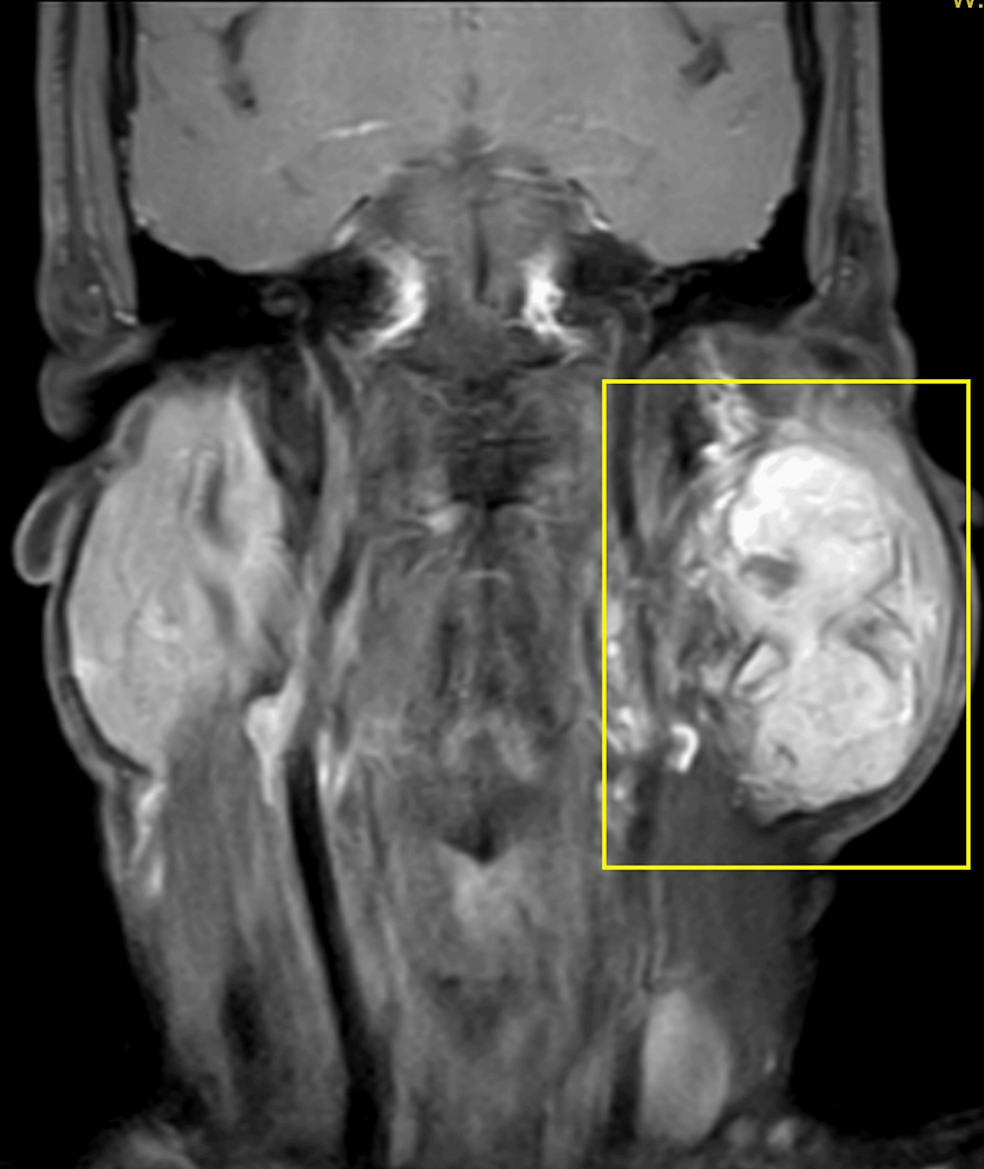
T1WI post-contrast sagittal MRI image Highlighted area showing the physical presentation of parotid gland tumor T1WI, T1-weighted image
